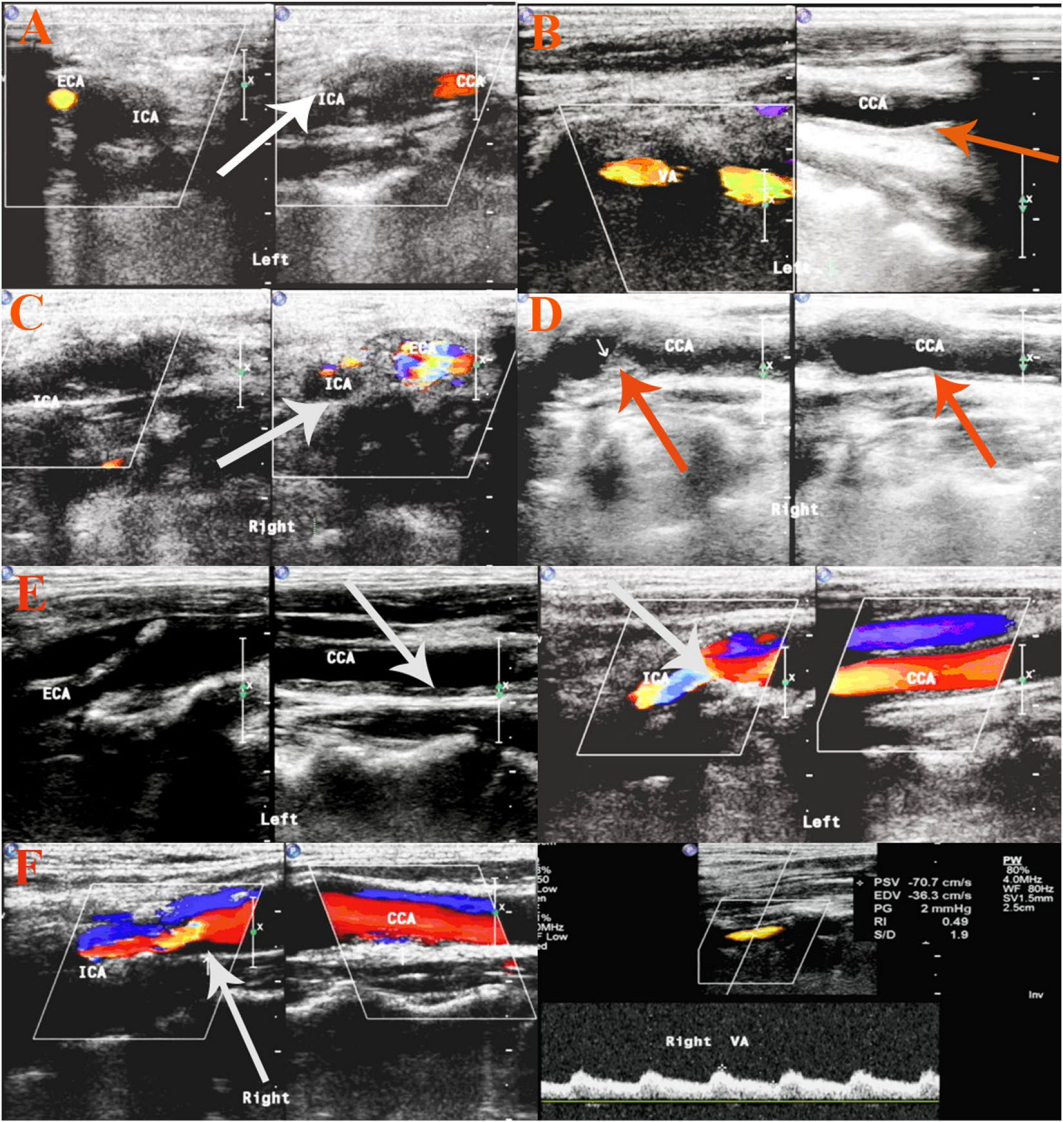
Representative carotid color duplex scan of two patients. (A–D) was the carotid ultrasonography of one patient. For common carotid artery (CCA) of the right side (R): intimal-medial thickness (IMT) was 1.4 mm; internal carotid artery(ICA): occlusion; For L-CCA: IMT 1.0–1.4 mm, resistance index (RI) 0.74; ICA: occlusion; R- vertebral artery(VA): D 3.9 mm, RI 0.50; L-VA: D 4.4 mm, RI 0.56; (E,F) is the other. R-CCA: IMT 1.2 mm, RI:0.64; ICA: IMT 1.1 mm, RI: 0.61. external carotid artery (ECA): IMT 1.3 mm, RI 0.67. L-CCA: IMT 2.0 mm, RI 0.8. ICA: IMT 1.3 mm, RI 0.66. R-VA: D 3.4 mm, RI 0.65. L-VA: D 4.0 mm, RI 0.60. Note the carotid artery (stenosis arrowheads).
